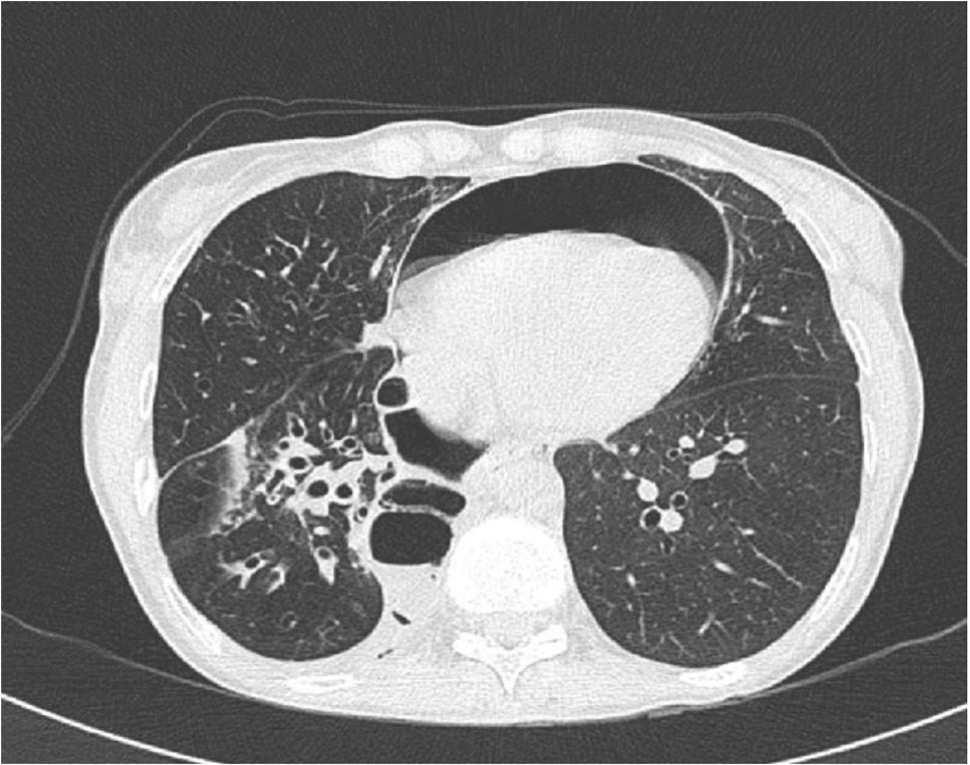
CT scan showing evidence of pneumopericardium consequent to the traumatic rupture of the right bronchial anastomotic line during attempted mechanical dilatation
